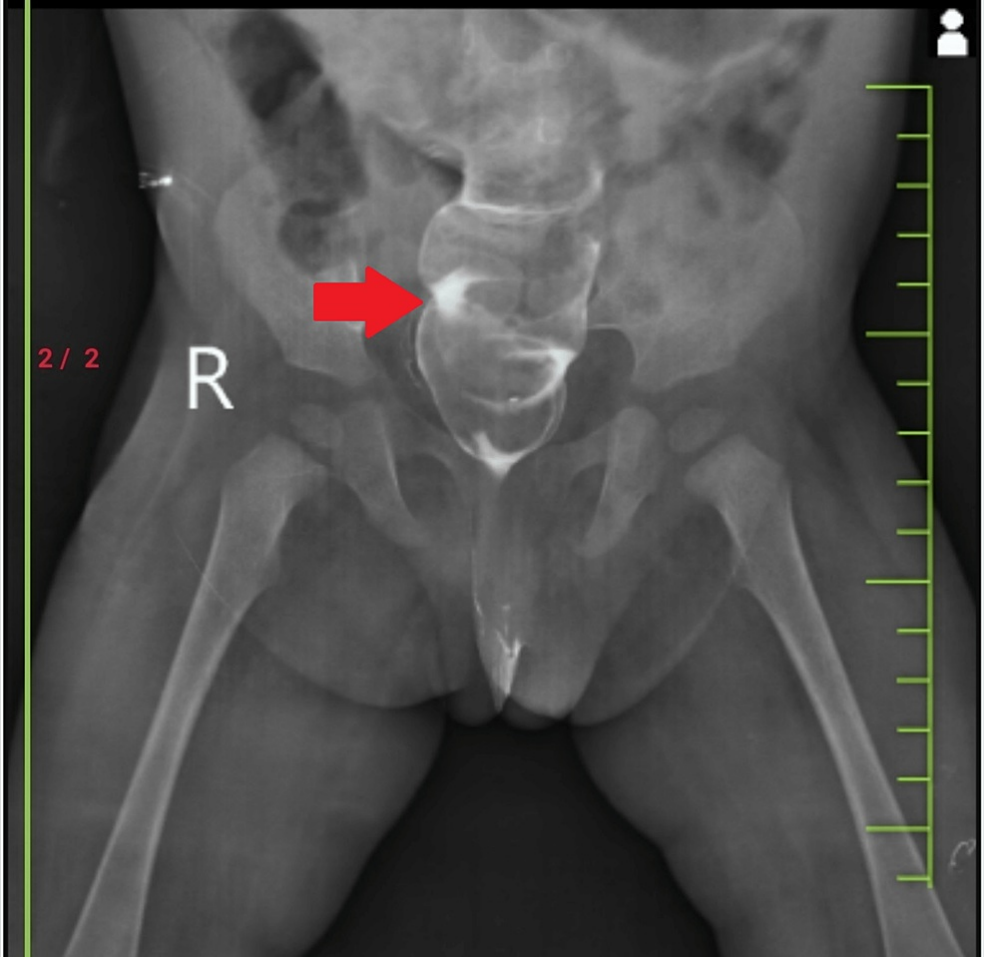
X-Ray lumbosacral spine anteroposterior view showing widening of the L2/L3,L4 vertebrae
